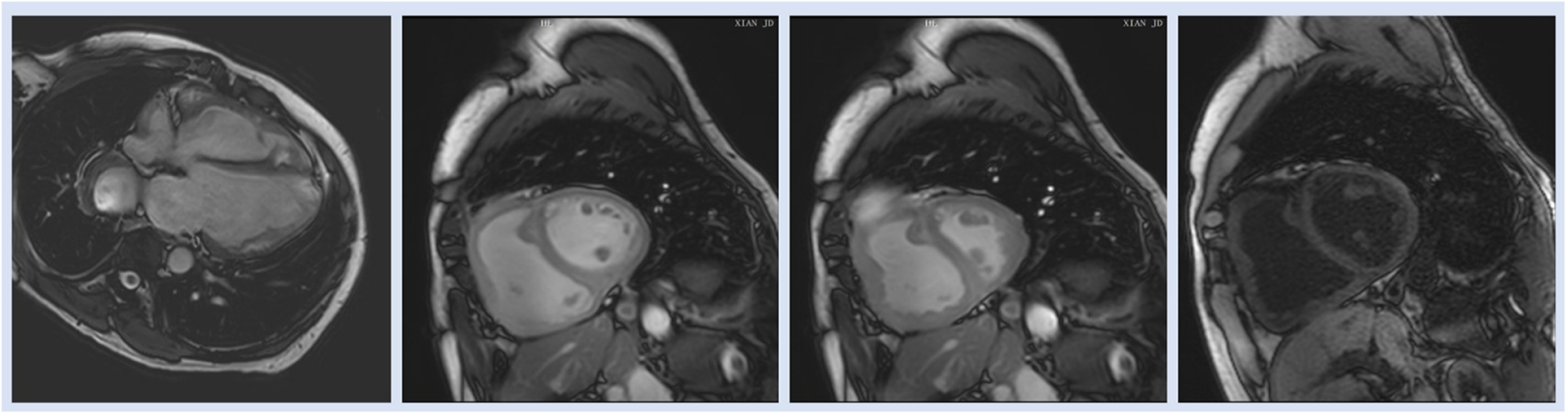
Representative images of cardiac magnetic resonance imaging in patient with PH.
